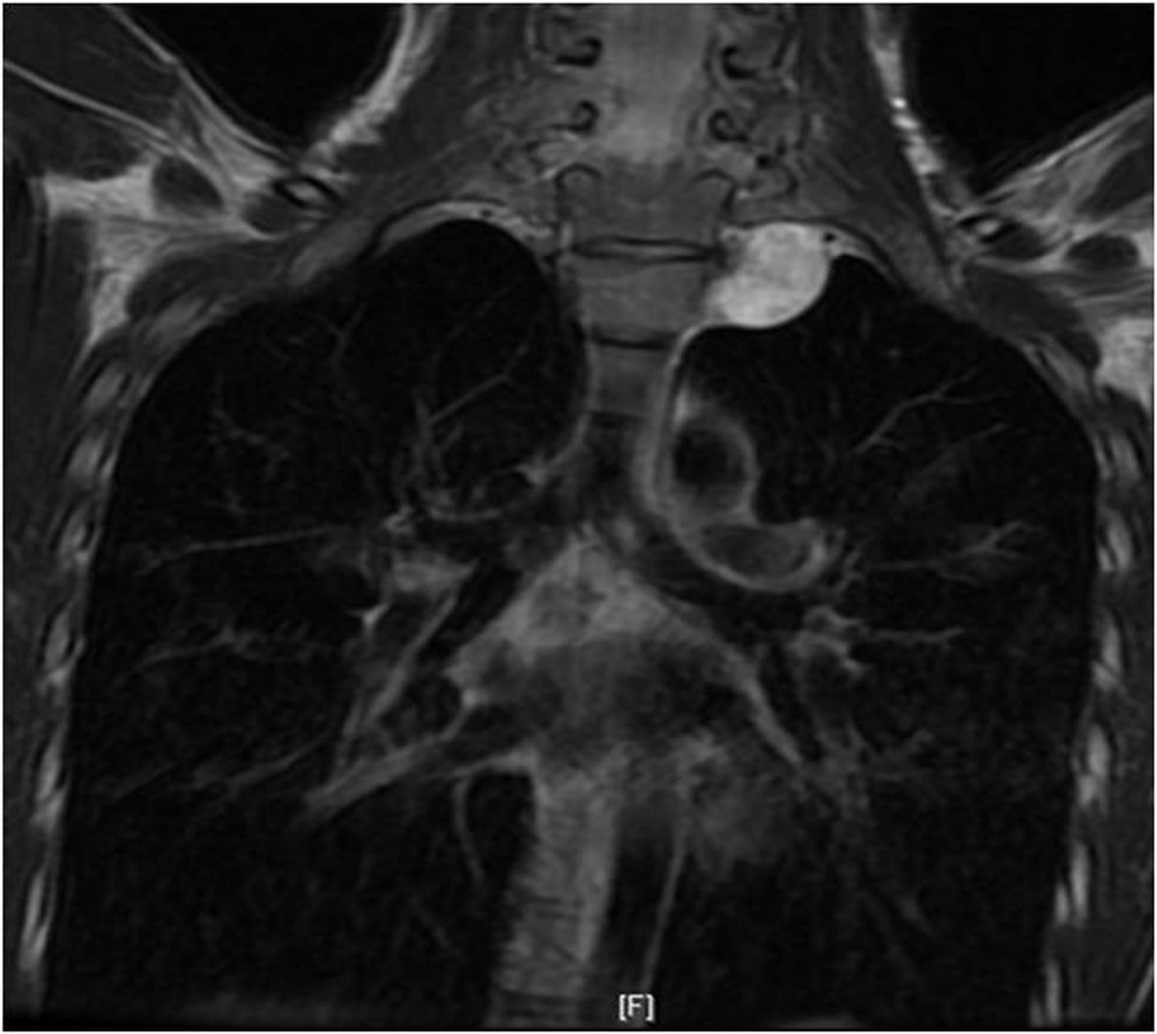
Chest magnetic resonance image (T2-weighted) revealing an inhomogeneous-intensity mass with no direct invasion of the left common carotid and subclavian arteries.
